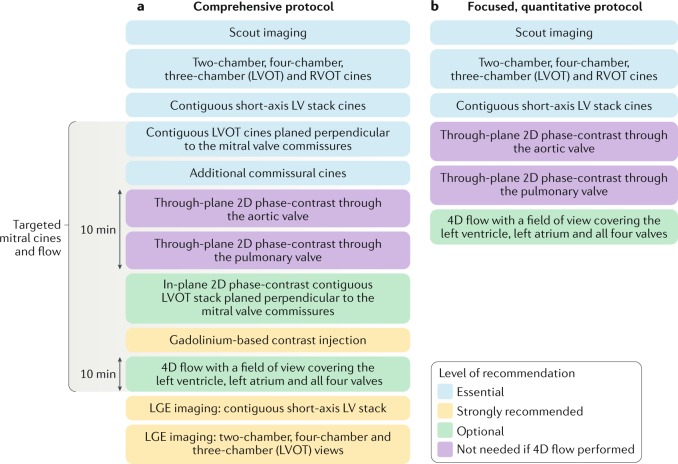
Recommended cardiovascular magnetic resonance imaging protocols for the assessment of mitral regurgitation. a | Comprehensive cardiovascular magnetic resonance imaging protocol for the assessment of mitral regurgitation. b | Focused, quantitative protocol. LGE, late gadolinium enhancement; LV, left ventricular; LVOT, left ventricular outflow tract; RVOT, right ventricular outflow tract.
